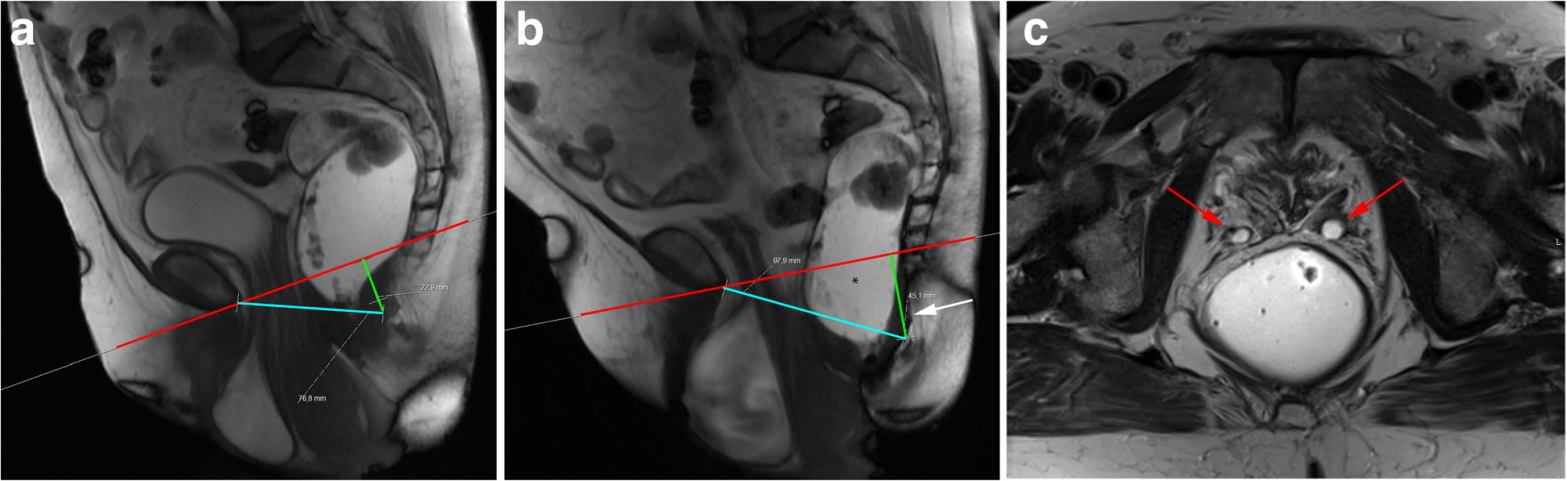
Descending perineal syndrome with tricompartimental prolapse. (a, b) Sagittal midline TruFISP images at rest (a) and during evacuation (b). H line (blue line) measures 7.7 cm (a) and 9.8 cm (b) and the M line (green line) measures 2.3 cm (a) and 4.5 cm (b), demonstrating widening and descent of the levator hiatus, even at rest but aggravating during defecation. It is also associated with tricompartimental prolapse, with a severe cystocele and a severe uterine everted prolapse. Note the verticalization of the levator plate (white arrow). Another interesting finding is that the rectal contrast is only partially expelled (b), because the mass effect by both the cystocele and uterine prolapse induces mechanical posterior obstruction (dilated rectum filled with ultrasound gel) causing constipation and incomplete evacuation (*). (c) Axial T2-TSE image at the pubic symphysis plane showing bilateral ureterohydronephosis causes by the cystocele (red arrows)
